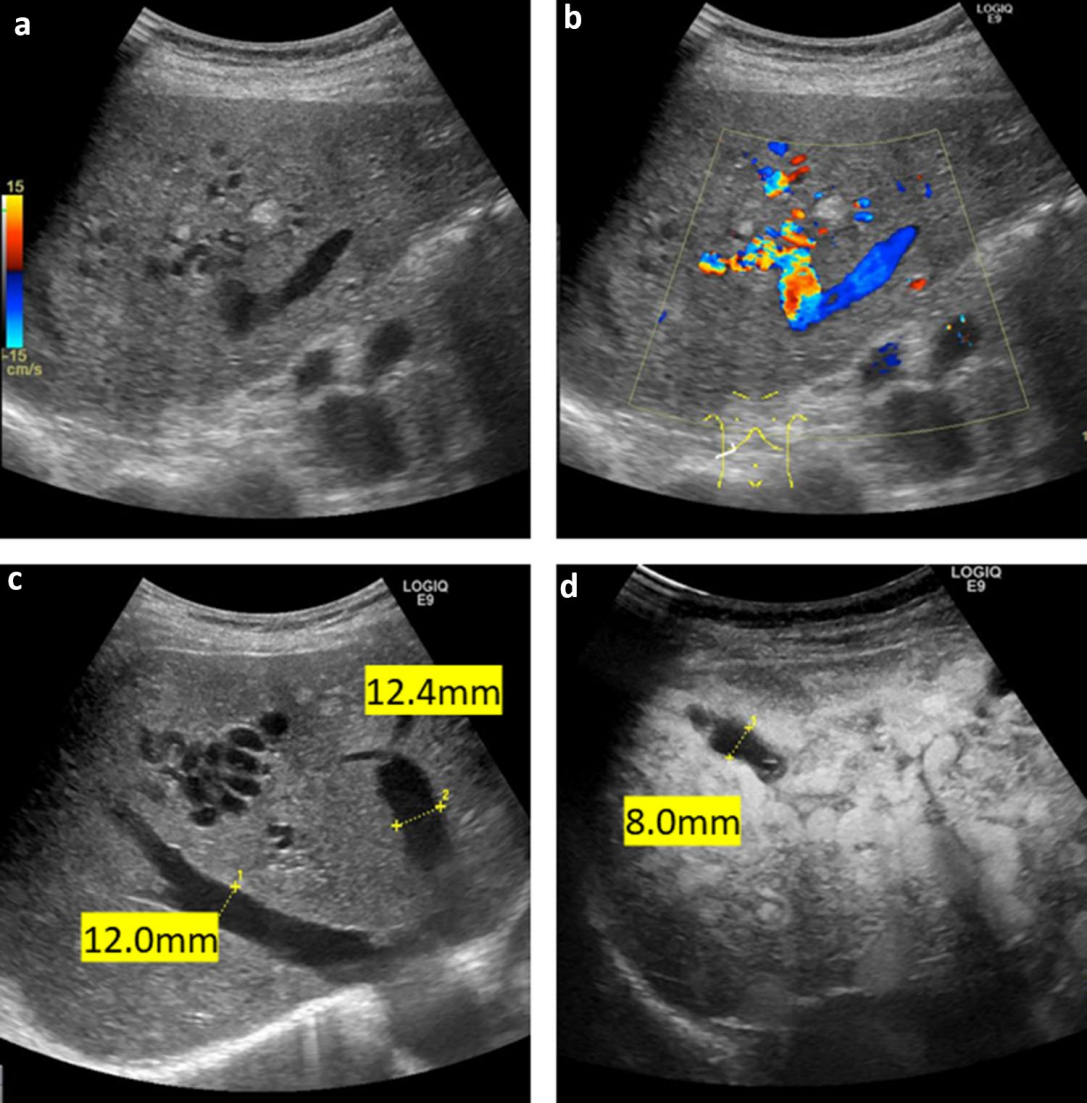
Abdominal ultrasonography (May 20XX + 3) a In the B-mode, arteriovenous anastomosis is observed in the right lobe of the liver. b Arteriovenous anastomoses are also observed on color Doppler imaging. cDilated and tortuous arteries are observed. Moreover, the right hepatic vein (RHV) appears significantly expanded by 12 mm and the middle hepatic vein (MHV) by 12.4 mm. d Contrast-enhanced ultrasound shows a bile duct dilated to 8 mm in liver S6.
